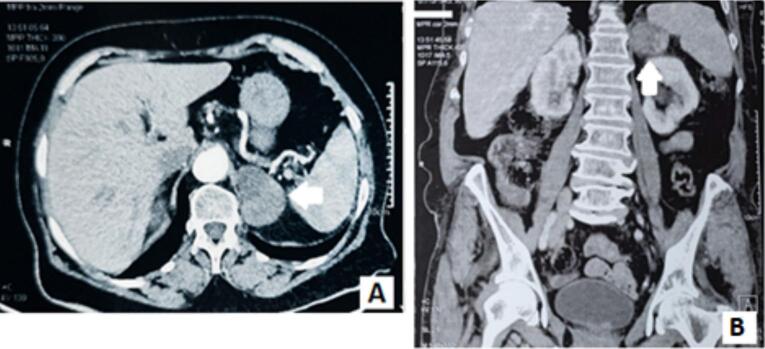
Enhanced abdominal CT-Scan showing a left suprarenal mass. Axial section (A) and frontal reconstruction (B) of the left adrenal mass at arterial and portal phase: showing heterogeneous enhancing of the lesion with low wash-out rate.
